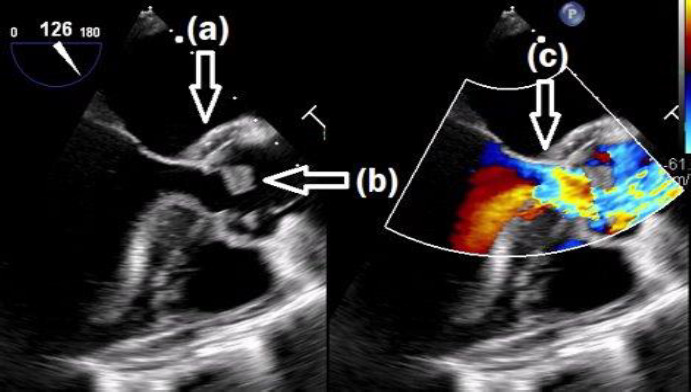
Echocardiogram image showing (a) root abscess, (b) vegetation and (c) aortic regurgitation.
